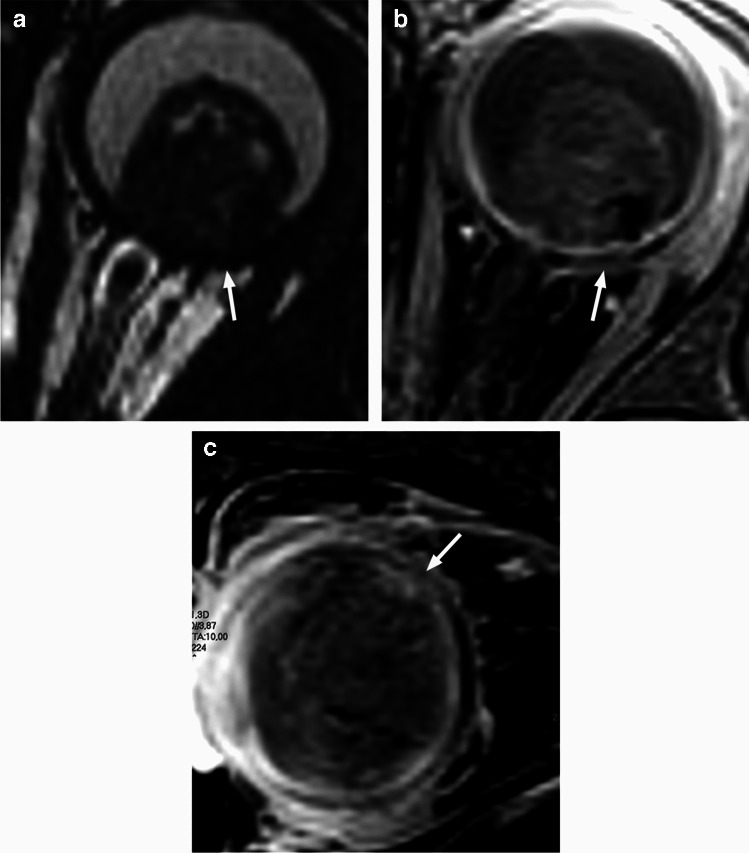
Retinoblastoma with scleral and extrascleral invasion. Axial T2-weighted image of the globe (a) shows a heterogenous mass with endophytic growth, consistent with retinoblastoma. The adjacent linear scleral hypointensity is irregular and discontinuous with extension of the mass into the retrobulbar fat (arrow). Corresponding postcontrast T1-weighted image (b) reveals enhancement of the affected sclera (arrow), which is confirmed on sagittal postcontrast T1-weighted image (c) demonstrating continuous enhancement of the intraocular lesion, sclera and retrobulbar fat (arrow)
